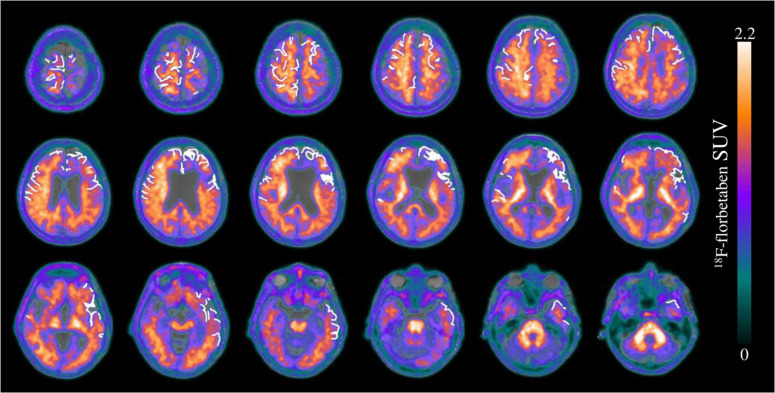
Transverse brain sections of an exemplary patient with cerebral amyloid angiopathy (CAA) (female, 75 years). The superimposition of cSS segmentation (white 0 and 1) and Aβ-PET (SUVr = 0–2.2) on a structural T1 magnetization prepared rapid gradient echo (MPRAGE) MRI sequence. The segmented cSS winded around the gyri and sulci as a linear structure. Since the patient was characterized by more than three affected sulci, cSS was classified as disseminated. A slight right-sided dominance was observed for the Aβ-PET signal, whereas the cSS distribution was left-sided predominantly in the frontal and the temporal lobe.
